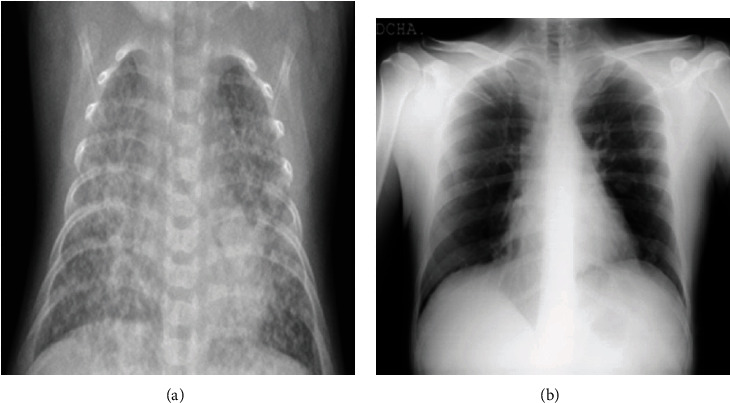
The sample X-ray images of (a) a viral pneumonia patient and (b) a COVID-19 patient as collected from the Kaggle database [46].
